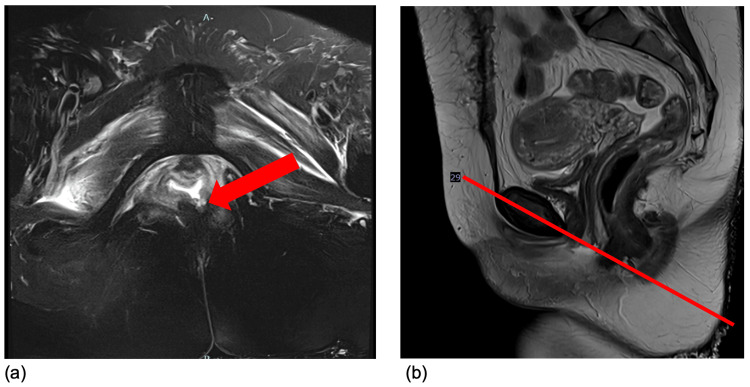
Magnetic resonance imaging (MRI) of the rectum for Case 2 Oblique (a) and sagittal (b) views of magnetic resonance imaging (MRI) of the rectum with and without contrast on hospital day 19, after four days of FMS use, showing fluid in the inferior vagina leaking into the rectum through the posterior wall towards the anal sphincter. Red arrow = area of defect. Red line = corresponding anatomical location of the fistula on sagittal imaging
